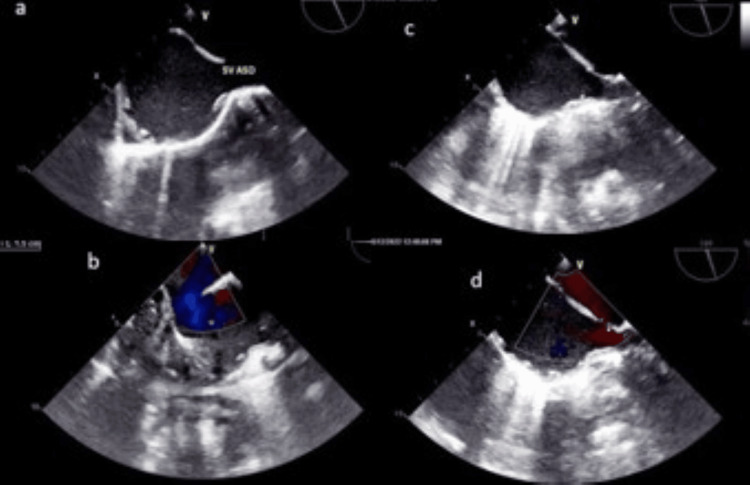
Transesophageal echocardiography. (a) Mid-esophageal bicaval view, pre-CPB 2D demonstrating SV-ASD.
